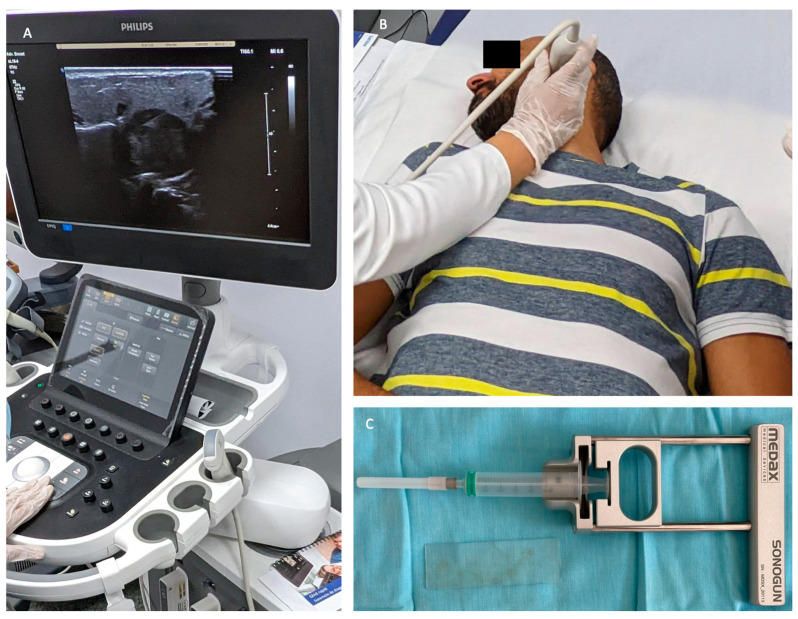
Ultrasound-guided FNAB for a left parotid tumor. Identification of the most representative tumor region for performing FNAB and ultrasound confirmation of the presence of the needle in the tumor (A,B). The gun-type device used to perform the puncture (C).
